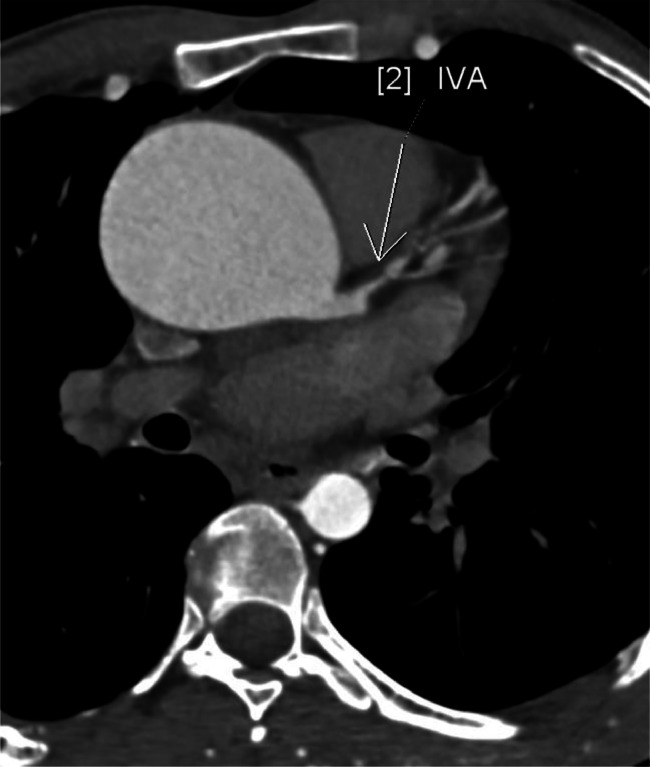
Oblique reconstruction CT image of the first segment of the IVA stenosis.
